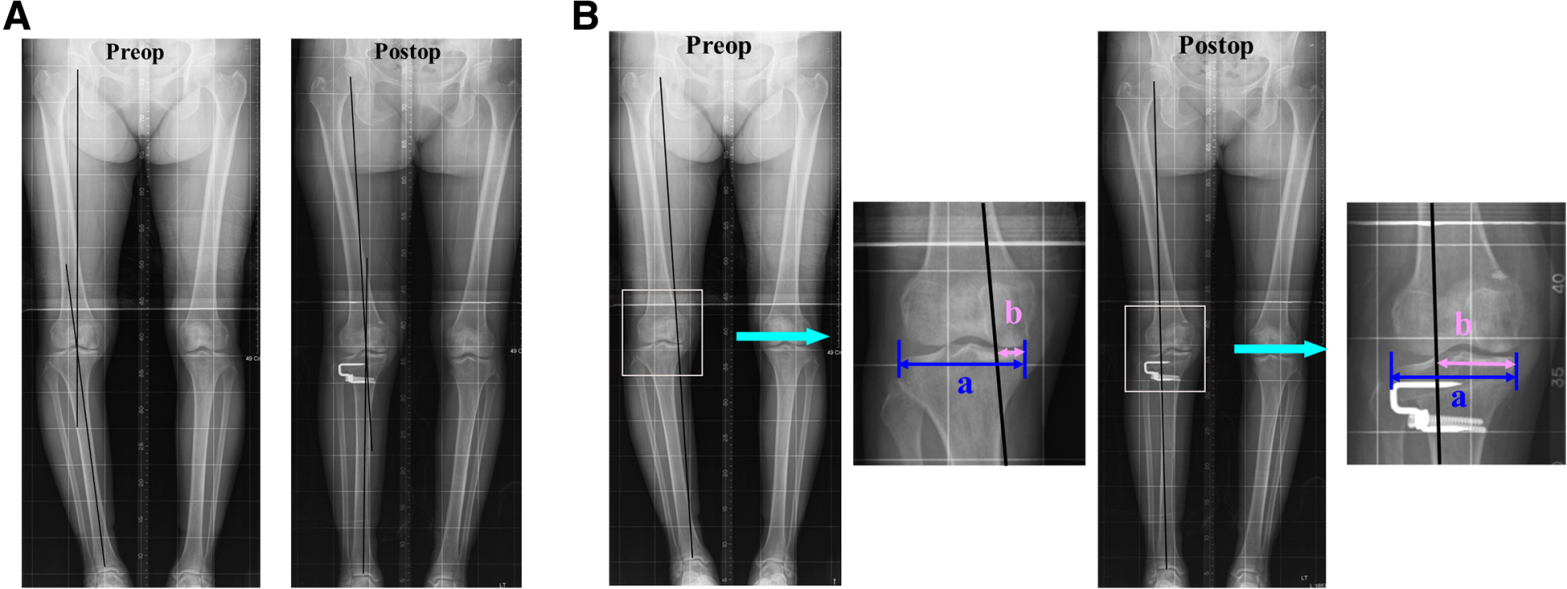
Radiographic measurement of the preoperative (preop) and postoperative (postop) mechanical axis (MA) and the percentage of the mechanical axis (MA%). a The MA was defined as the angle between the femoral and tibial mechanical axes on an orthoroentgenogram. b The MA% shown on the orthoroentgenogram was evaluated by percentile denotation ([b/a] × 100), where a is the width of the tibia plateau and b is the distance from the medial border of the medial tibial condyle to the point at which the mechanical axis intersects the knee joint line
